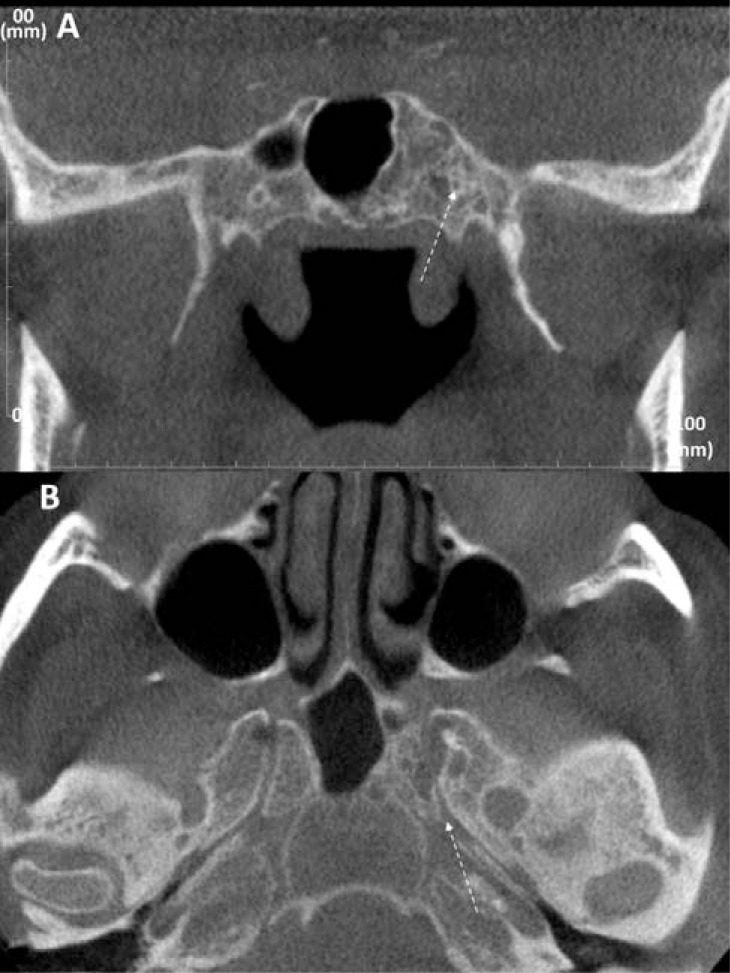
Example of arrested pneumatization of the sphenoid (APS) affecting the vidian canal. The left vidian canal (*dashed arrow*) is smaller in (A) coronal CBCT image and irregular in (B) axial CBCT image than the right canal, but the cortical outline of the canal is largely maintained (male, 61 years).
